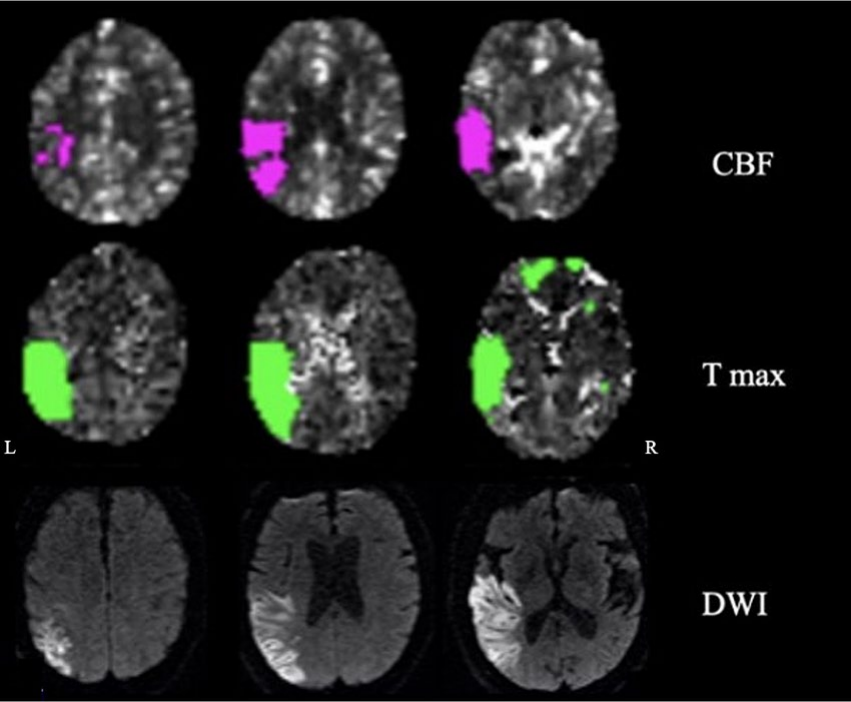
Neuroimaging: cerebral blood flow (CBF), time-to-maximum (Tmax), and diffusion-weighted imaging (DWI) showing penumbra and ischemic regions.
